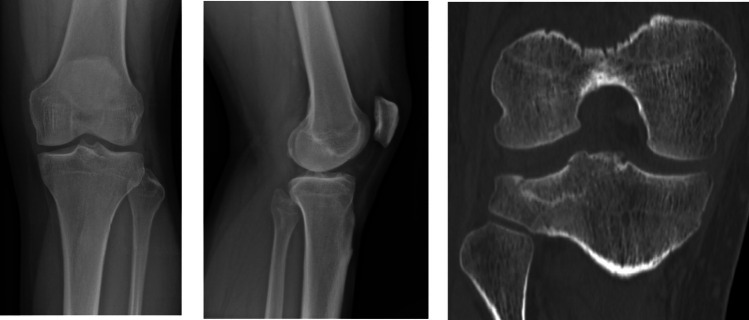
Left knee: tibial plateau occult fracture not detected on X-ray (left and middle images) and later identified on CT (right image)
